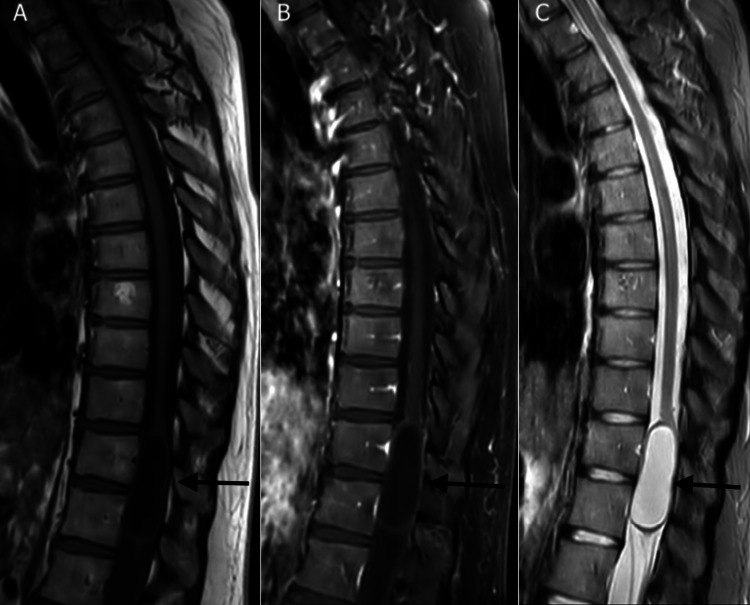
Sagittal MRI thoracic spine redemonstrating cystic lesion that has increased in size on follow-up imaging nine years later. (A) T1 non-contrast sequence; (B) T1 post-contrast; (C) T2.
